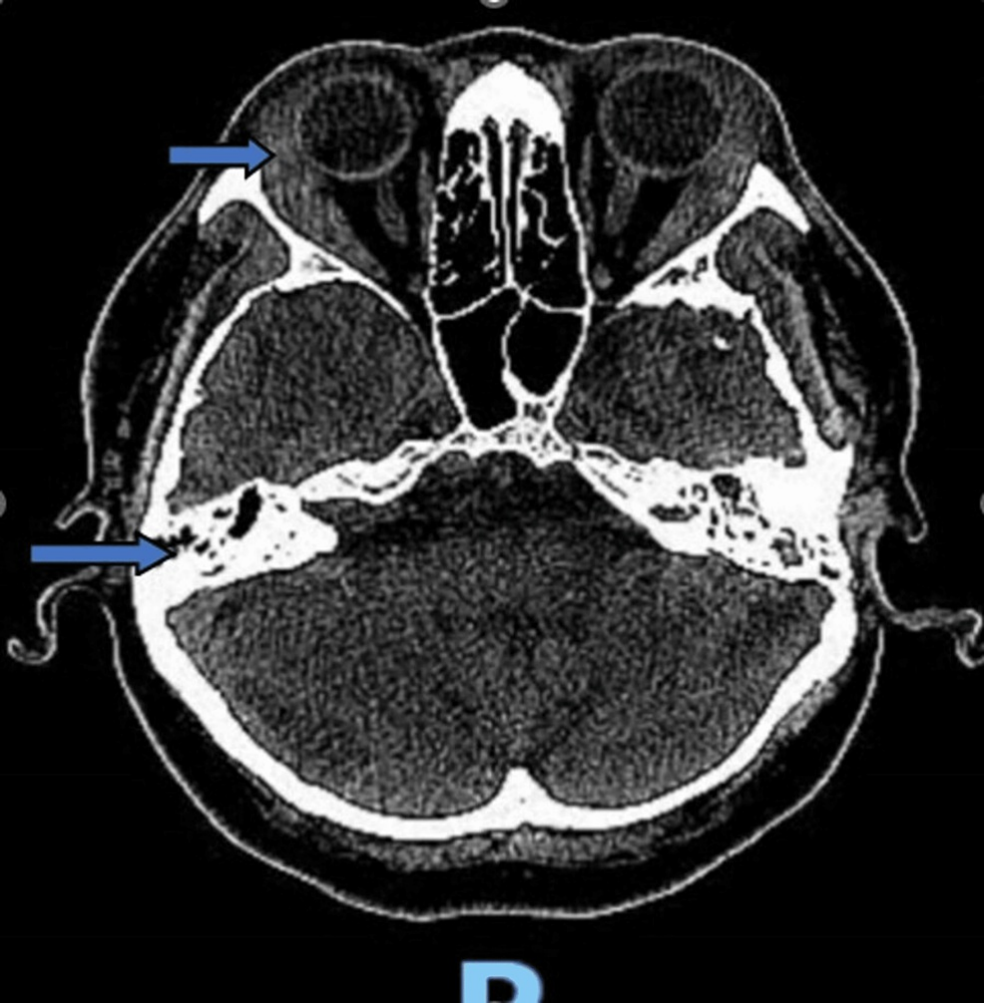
CT of the head with lacrimal hyperplasia and mastoid air cell opacification.
